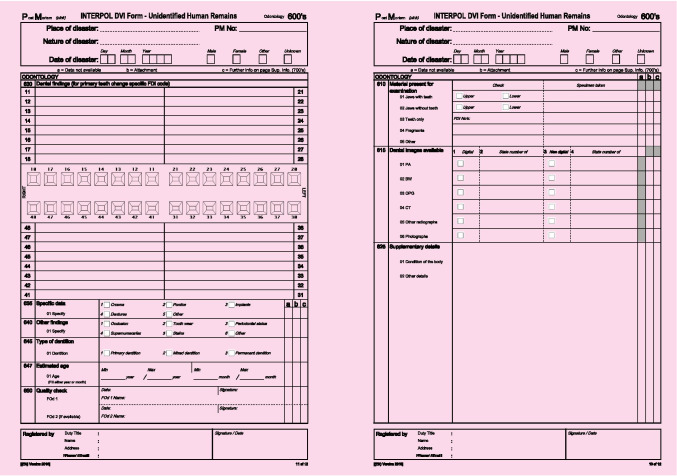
a PM information package as presented for the students; newly performed bitewing radiographs, intraoral pictures, video clips of the IOS scan. b Interpol form, disaster victim identification, unidentified human remains postmortem.
